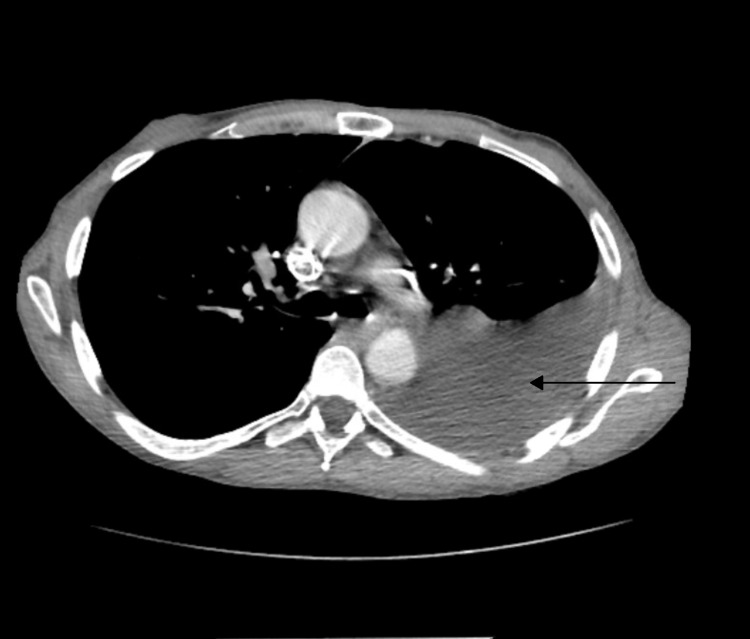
Chest CT shows left pleural effusion (arrow).
